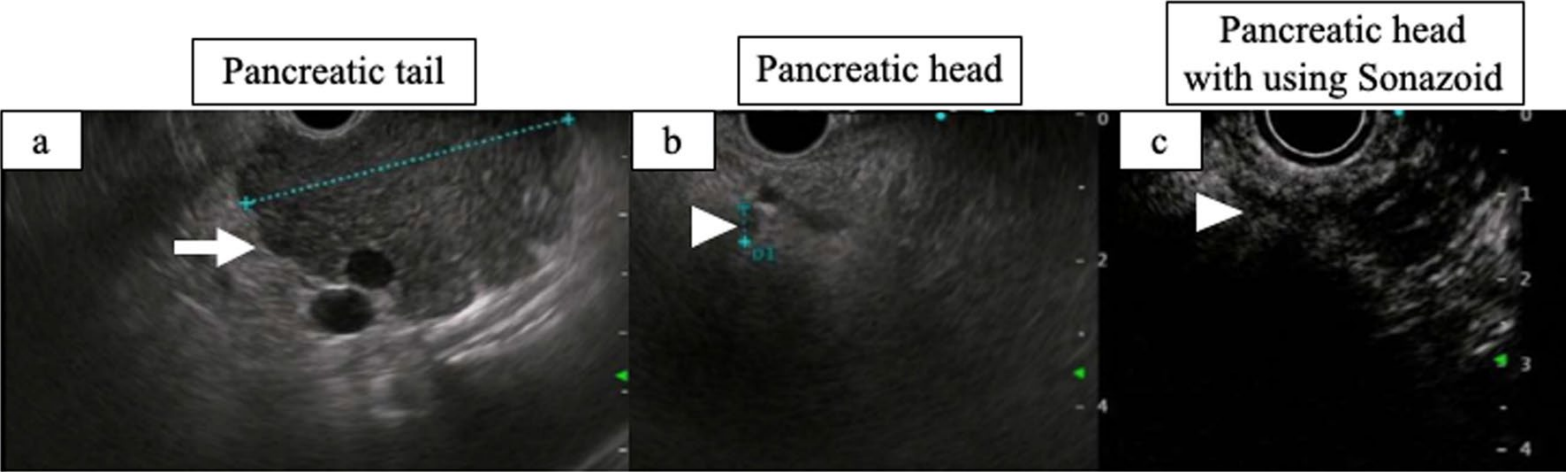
Endoscopic ultrasonography. a A hypoechoic lesion, 55 mm in diameter, is detected in the pancreatic tail (white arrow). b A hypoechoic lesion, 5 mm in diameter, is detected in the pancreatic head (white arrowhead). c A hypoechoic lesion is not enhanced with Sonazoid (white arrowhead)
